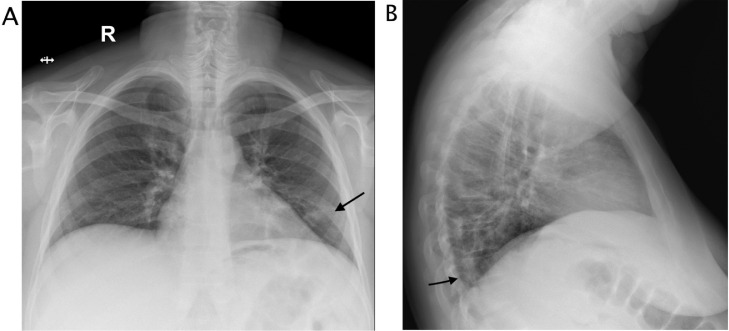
Chest X-Ray in posteroanterior (A) and lateral (B) projections. On the left basal lung, a small area of infiltrate is appreciable (black arrow). The diaphragm is well defined, no pleural effusion is detected.
